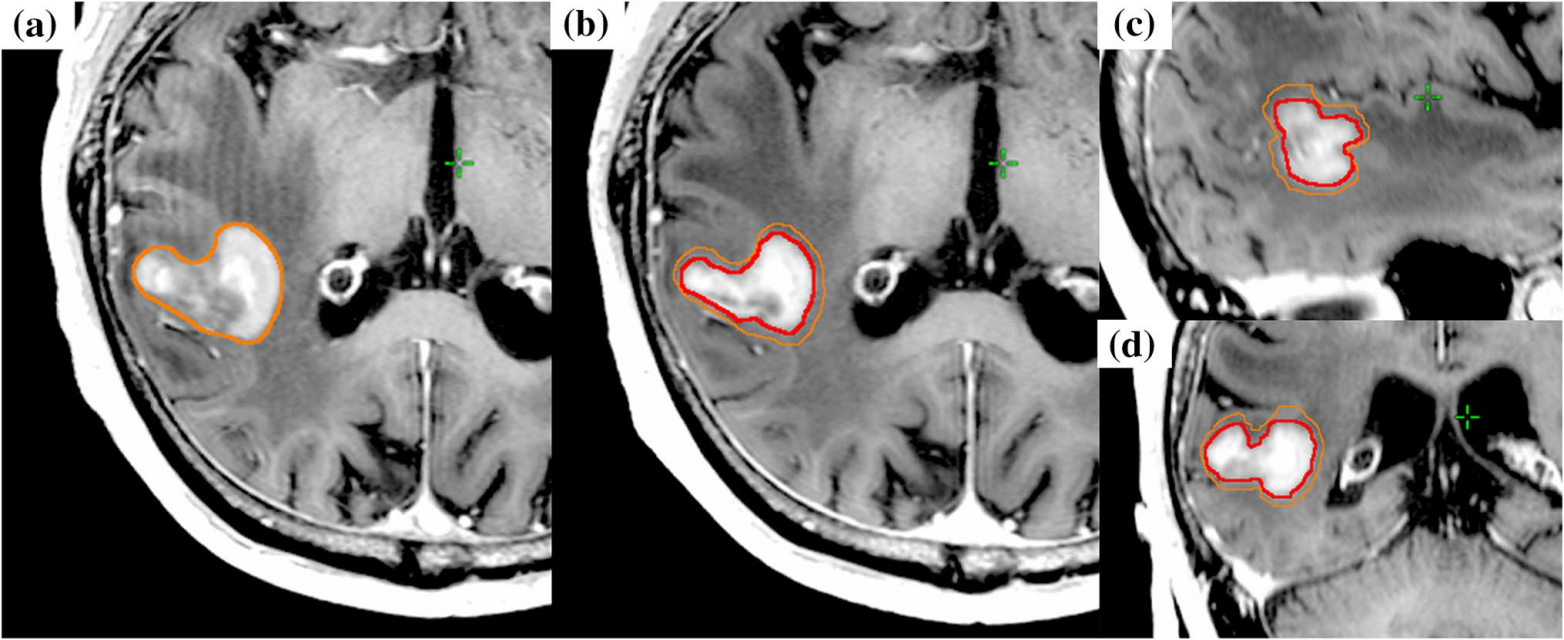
The case of tumor volume reduction during stereotactic radiotherapy. The case was brain metastasis of which primary tumor was colon adenocarcinoma. Orange line shows the gross tumor volume (GTV) of the initial plan, and red line shows GTV at the modification. a Axial: T1-weighted magnetic resonance imaging (MRI) with gadolinium (Gd) of the initial plan. b, c, d Axial, Sagittal, Coronal: T1-weighted MRI with Gd before 3rd treatment for the modification (the fifth day of SRT start)
